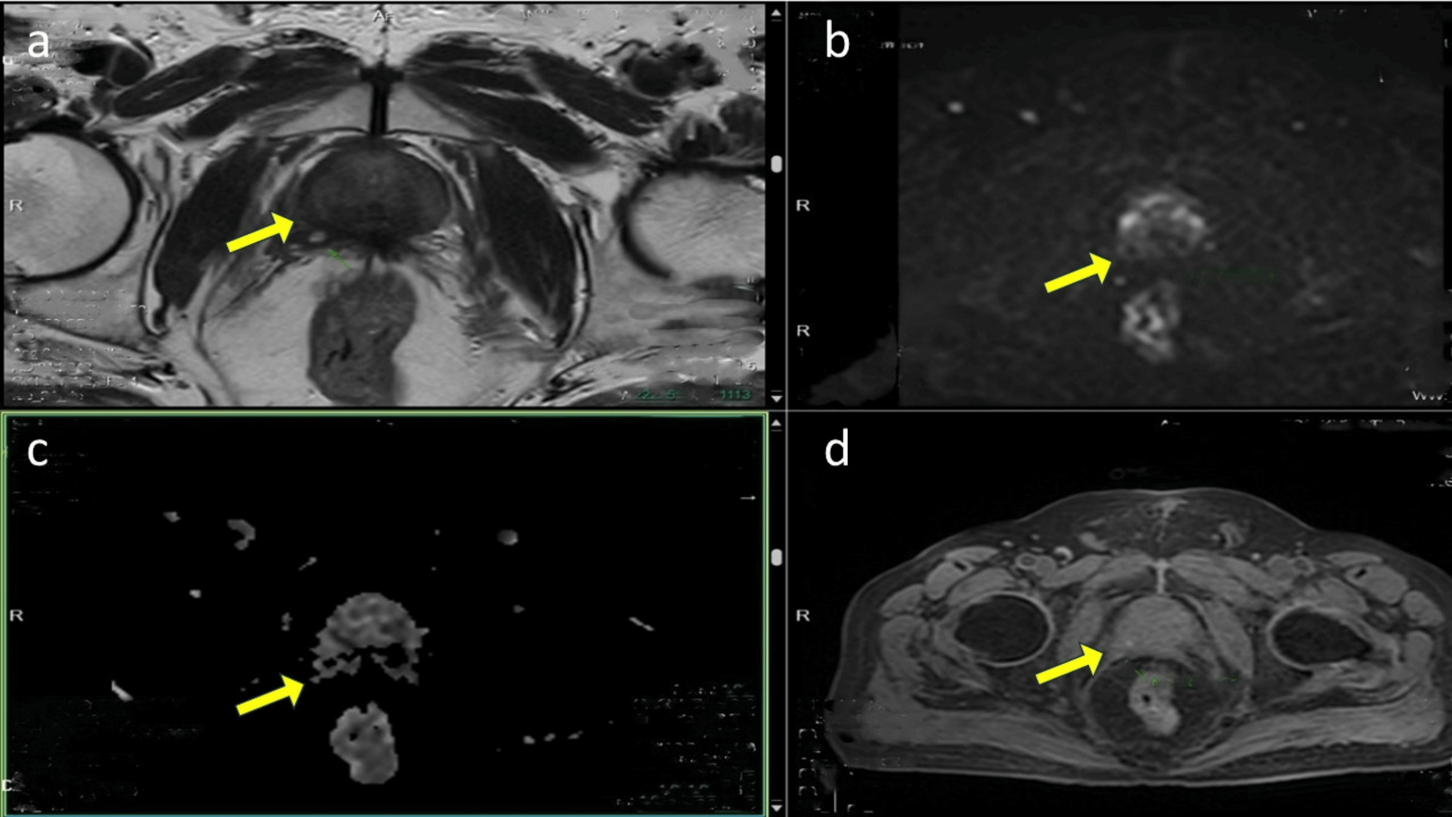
Post-ADT MpMRI prostate a: Ill-defined T2 hypointensity in the right posterolateral aspect of the peripheral zone in mid-body extending to the apex, representing an area of post-treatment scarring/desmoplastic reaction. b and c: The prostatic lesion showed no evidence of diffusion restriction/ADC hypointensity or differential focal enhancement. Fat planes with adjacent levator ani muscles appeared well-maintained. No evidence of enlarged pelvic lymph nodes. d: The periprostatic-perivesical fascia were unremarkable. There was no free fluid in the pelvis. The pelvic side walls were free, and the bony pelvis was unremarkable. Features were suggestive of a PRECISE score of 2. ADT: androgen deprivation therapy; MpMRI: multiparametric magnetic resonance imaging; ADC: apparent diffusion coefficient; PRECISE: Prostate Cancer Radiological Estimation of Change in Sequential Evaluation
